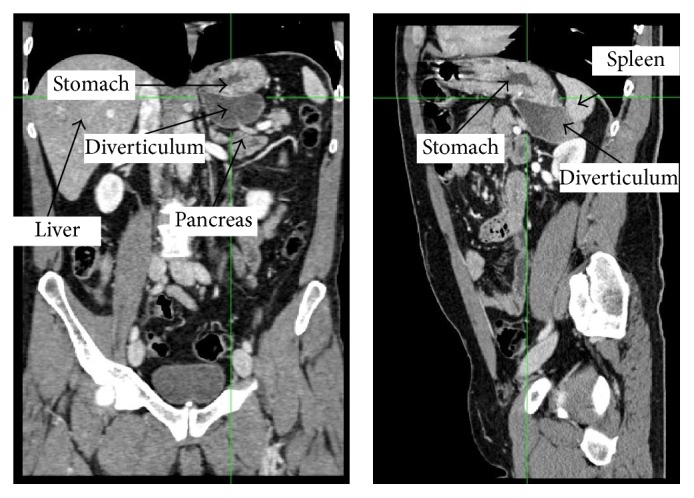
Arterial phase CT scan (frontal and sagittal planes) showing a large diverticulum of the size of 52 × 68 × 72 millimeters protruding from the gastric fundus, with fluid content. The retroperitoneal location of the pouch is well visible in the sagittal scan, as well as its tight adhesions with the inferomedial surface of the spleen, the ipsilateral adrenal gland, and the upper posterior surface of the body and tail of the pancreas.
